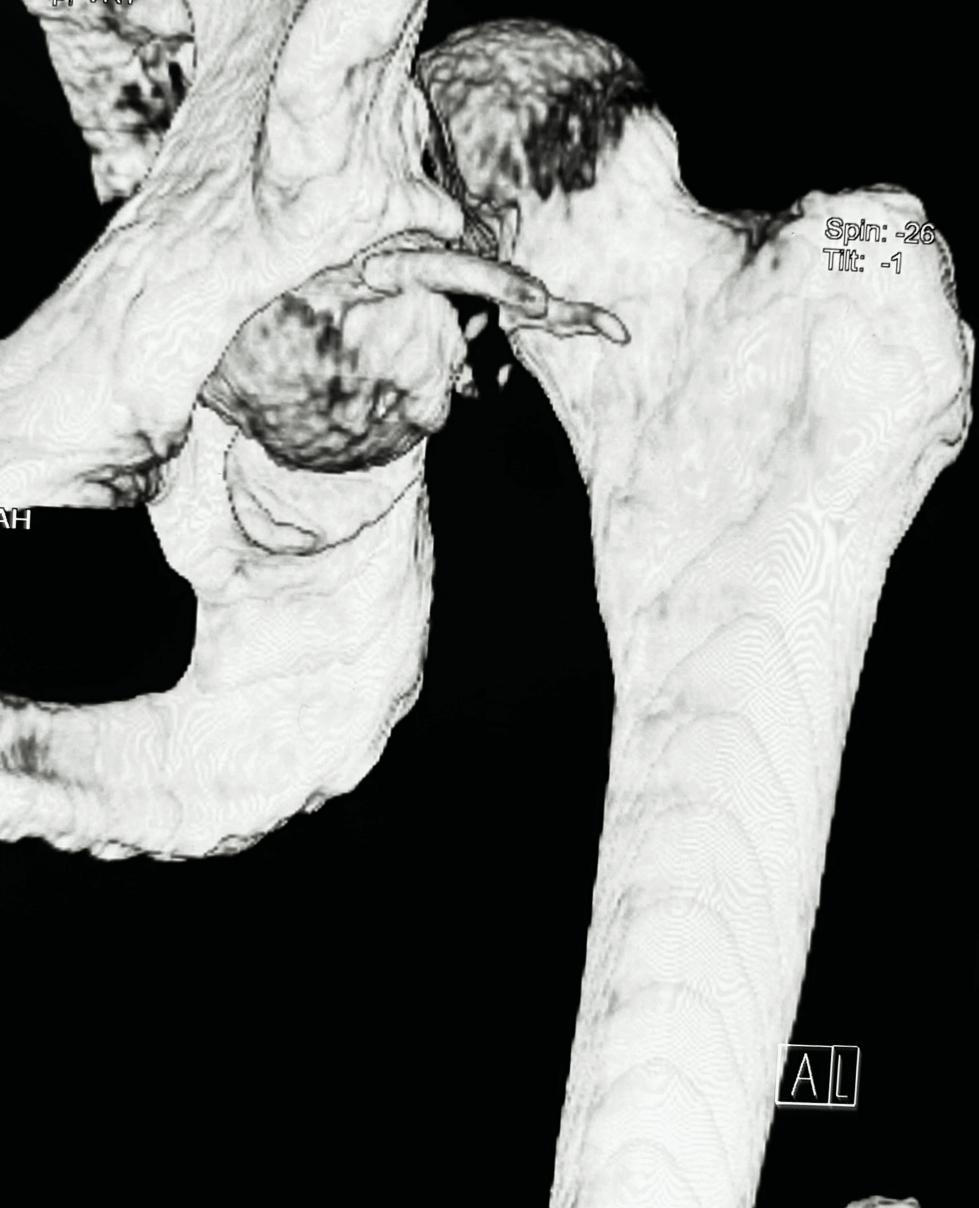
Computed tomography (CT) scan with 3D reconstruction delineating femoral head fragment size and multiple loose fragments of case no 1
